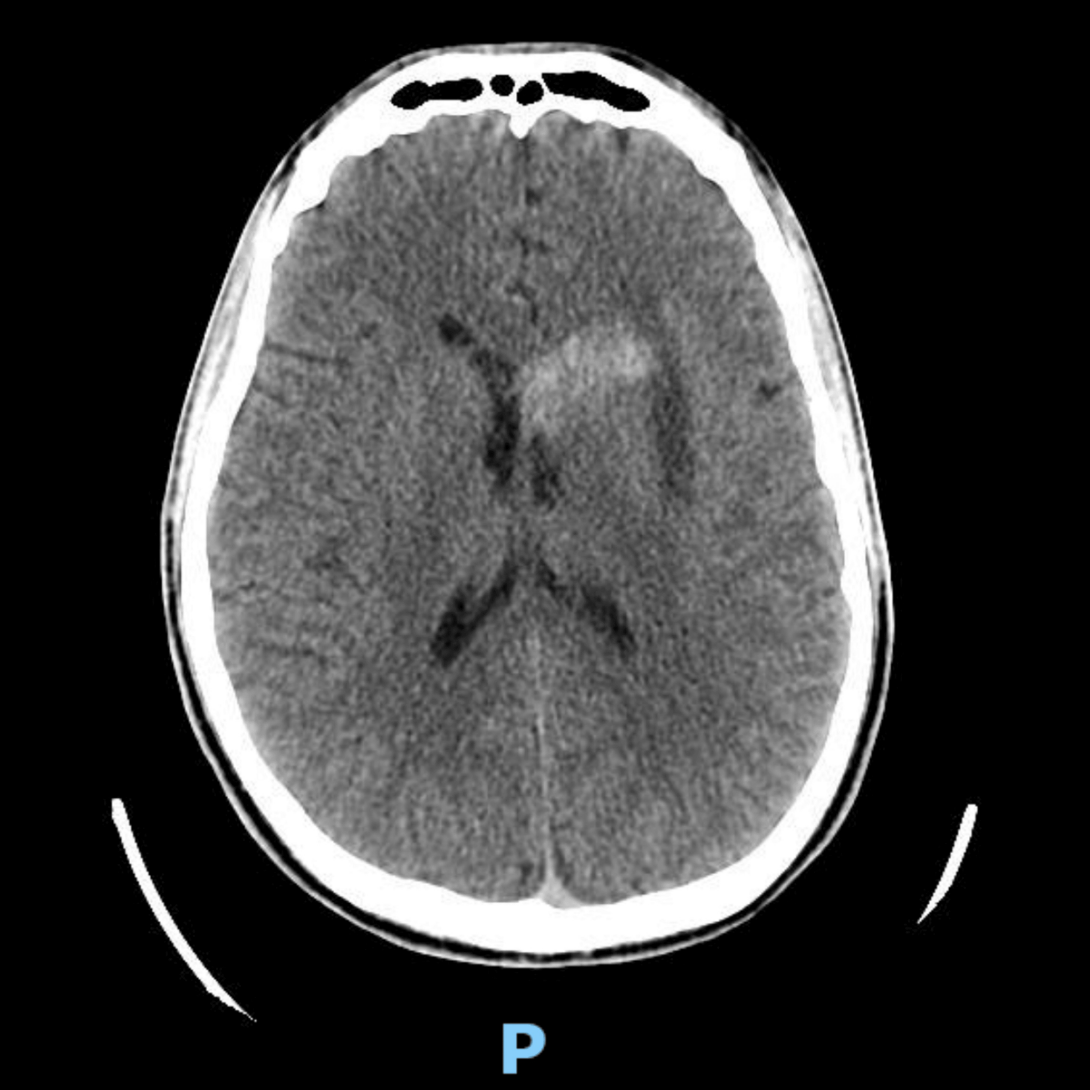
Head CT Non-contrast axial CT of the head showing a hyperdense hemorrhagic transformation of an acute infarct in the left basal ganglia with extension into the lateral ventricle, consistent with decompressive intraventricular hemorrhage.
